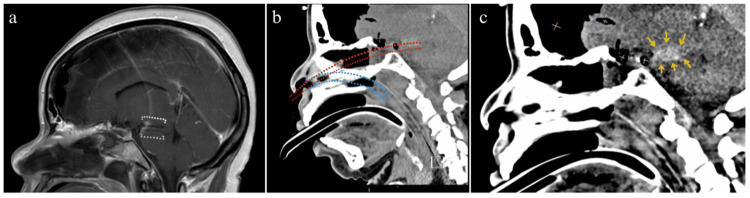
(a) MRI brain, sagittal view showing pontine hemorrhage (between dotted lines). (b) CT showing the path that the NGT travelled through the superior pons (red), and the planned NGT path (blue). (c) CT showing pontine hemorrhage (arrows), and pneumocephalus along the frontal convexities (yellow X) NGT, nasogastric tube.
